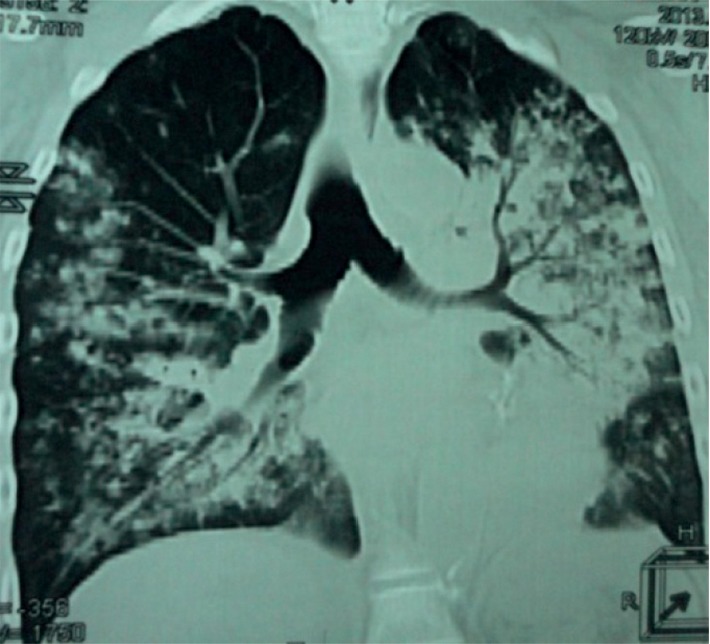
Chest CT: bilateral alveolar infiltrates in a ground-glass pattern.
